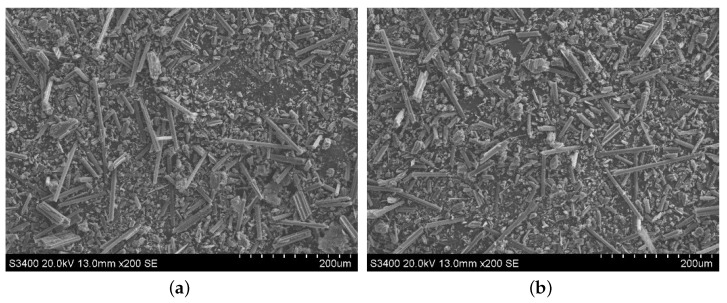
Morphologies comparison when $a_{p}$ is 0.1 mm. (a) is ordinary cutting and (b) is ultrasound- assisted cutting.
